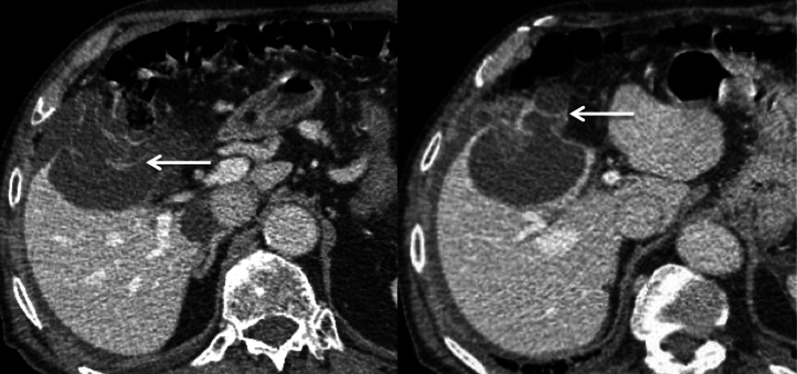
Rupture of a hepatic cyst in a 89-year-old male with severe abdominal pain. Axial computed tomography on portal venous phase demonstrates a floating wall (arrow) associated with pericystic and peri-hepatic fluid collection
